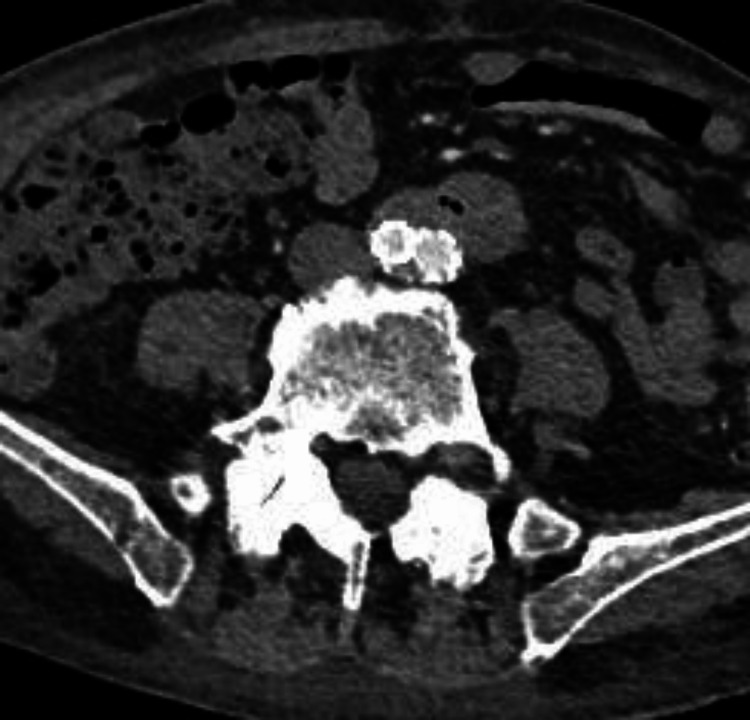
Postoperative follow-up with abdominal CT angiography showed no complications in the intervention site.
